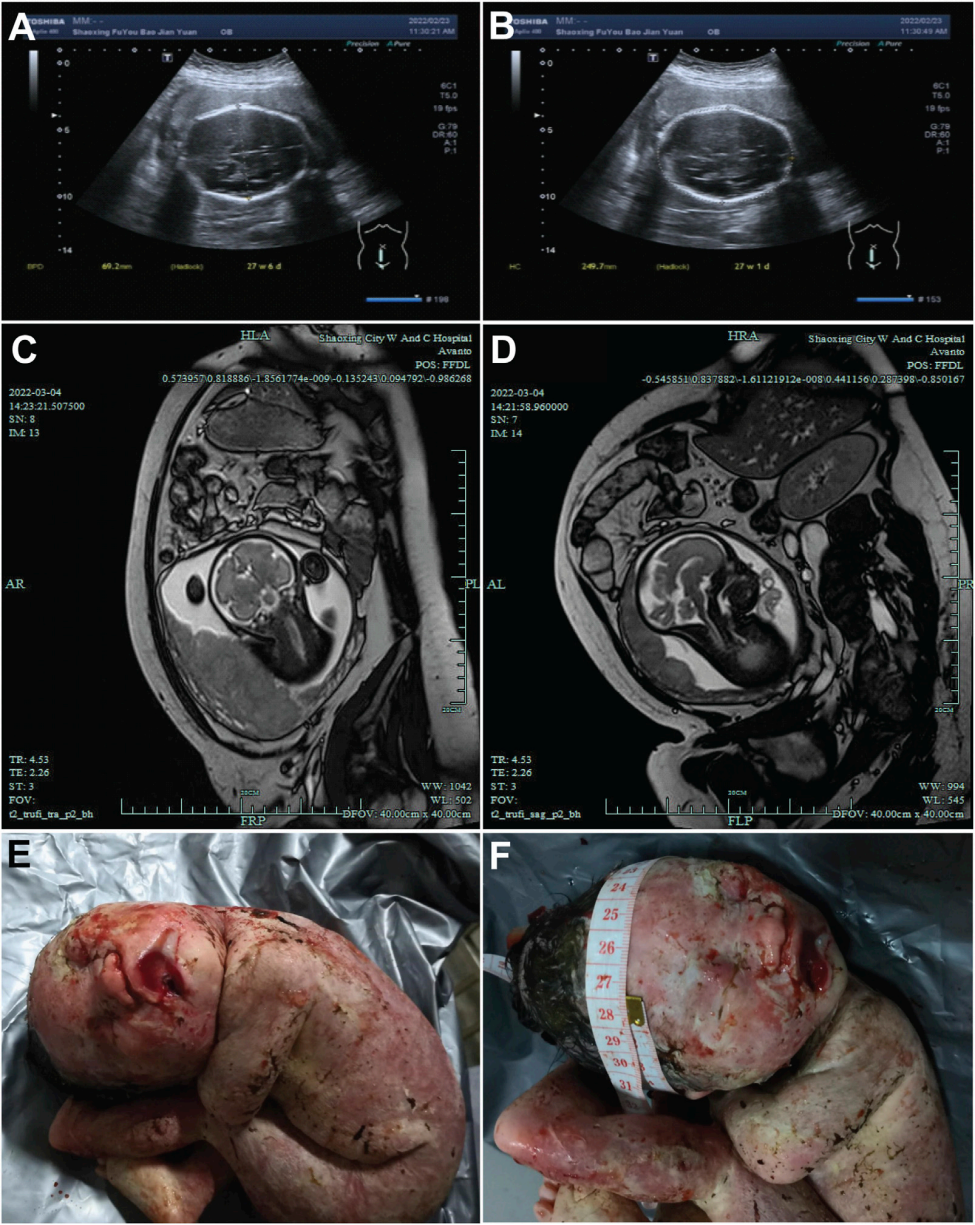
Ultrasound and magnetic resonance images of the fetal head. (A,B) Ultrasound images of the fetal head at 27 weeks + 4 days of gestation, showing the fetal head from different sections. The measured values indicated in the figures (such as 46.2 mm and 240.7 mm) represent the relevant diameters of the fetal head in the corresponding sections. (C,D) MRI images of the fetal head at 27 weeks +4 days of gestation, presenting the structure of the fetal head from different angles. (E,F) Post-induction labor photographs of the fetus. E presents the general appearance of the fetus. F focuses on the fetal head, where a measuring tape is used to document the head circumference.
